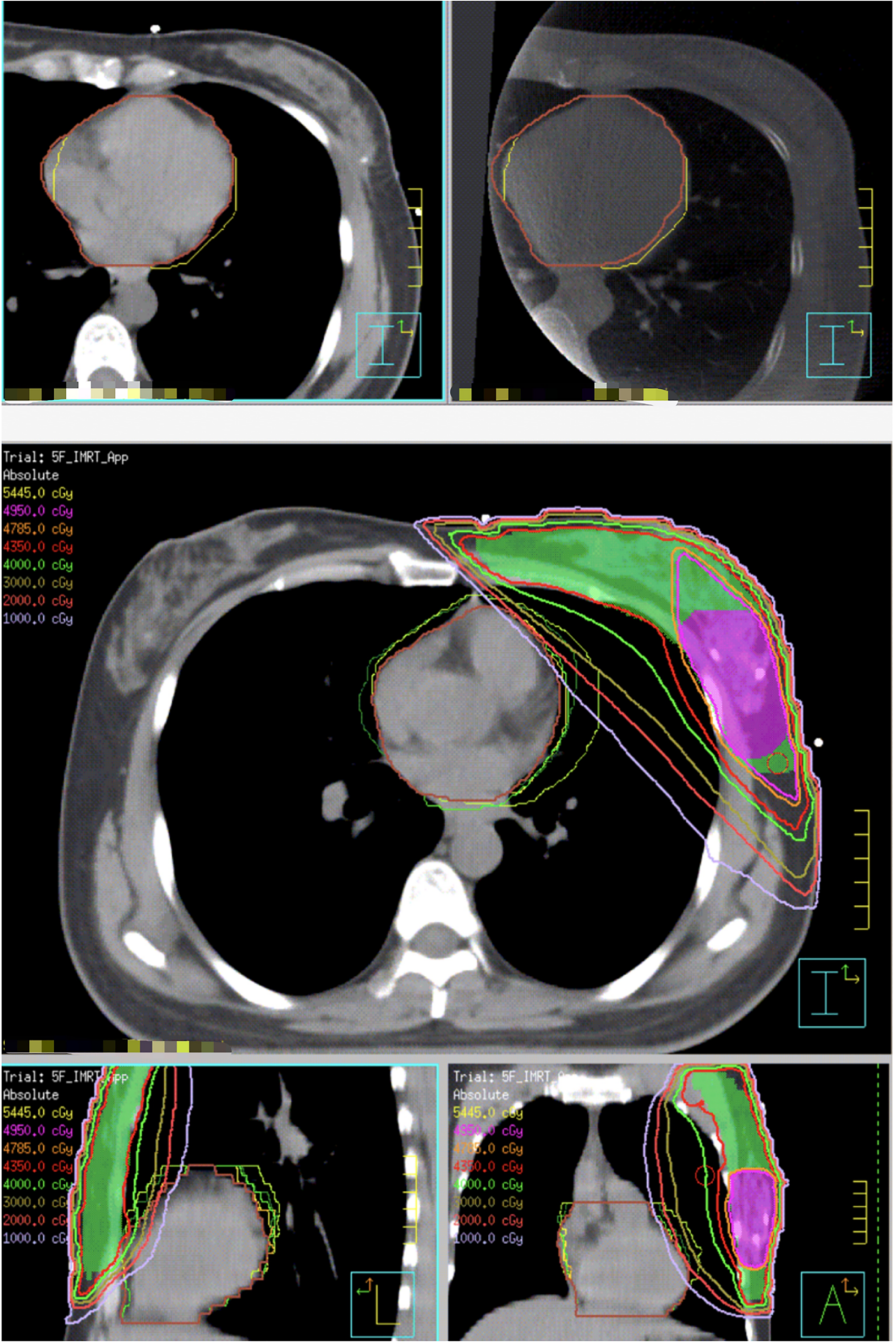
(A, upper left) Heart contour on the voluntary deep inspiration breath-hold (VDIBH)-planned computed tomography (CT) (brown); (B, upper right) heart contour on cone beam computed tomography (CBCT) (yellow); (C, lower middle) all heart contours from CBCT images registered to the voluntary deep inspiration breath-hold (VDIBH)-planned CT of one patient for heart position and heart dose evaluation.
